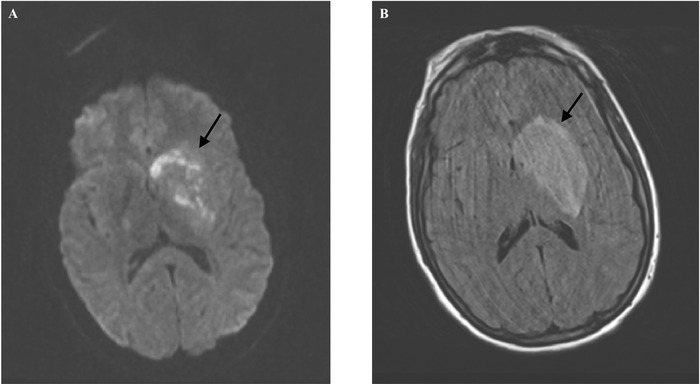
Mass-like lesion centered in the left basal ganglia extending to the left crus cerebri (arrows) is shown in (A) magnetic resonance imaging (MRI) brain diffusion weighted imaging (DWI) with contrast and (B) axial view MRI brain T2-weighted fluid-attenuated inversion recovery (FLAIR).
